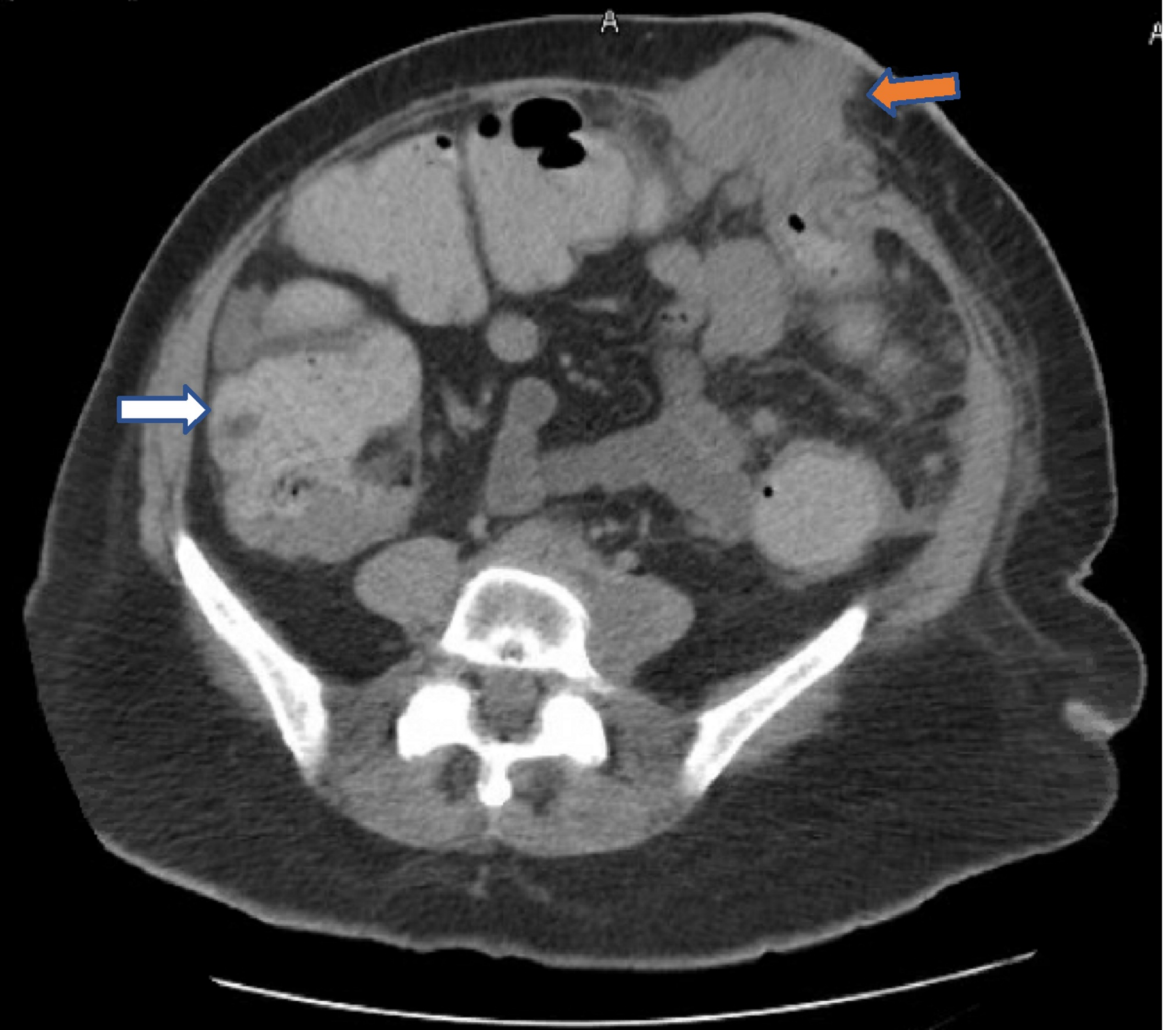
Axial cuts of an abdominal CT scan showing obstruction at the level of the colostomy by a mass (orange arrow), resulting in significant proximal colonic dilation (white arrow)
